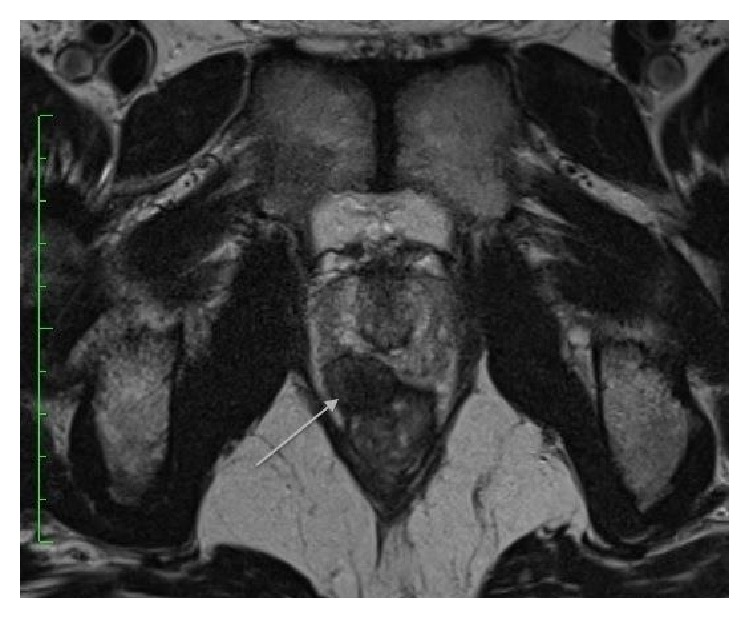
T2-weighted axial MRI at the level of the pubic symphysis demonstrating a T2 low signal intensity lesion adjacent to the right peripheral zone of the prostatic apex measuring 1.7 × 1.4 cm (arrow).
